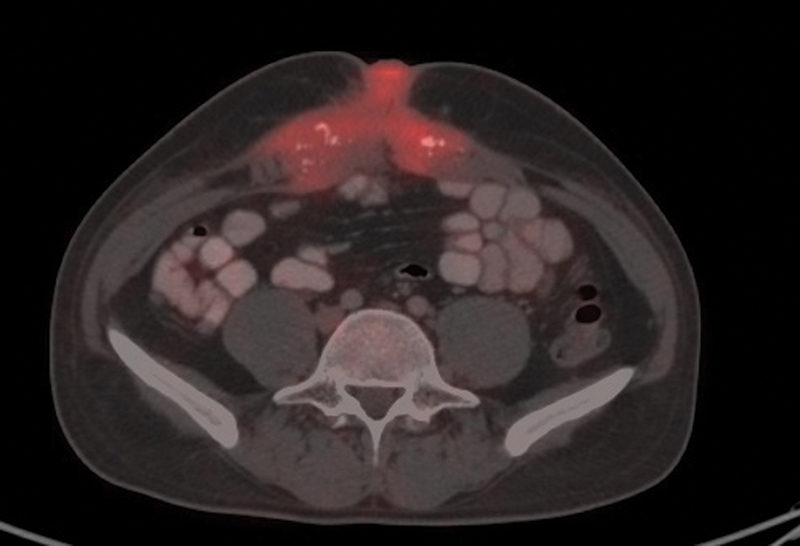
PET CT scan showing nodular lesion at umbilicus and omental nodules in sub hepatic region.
